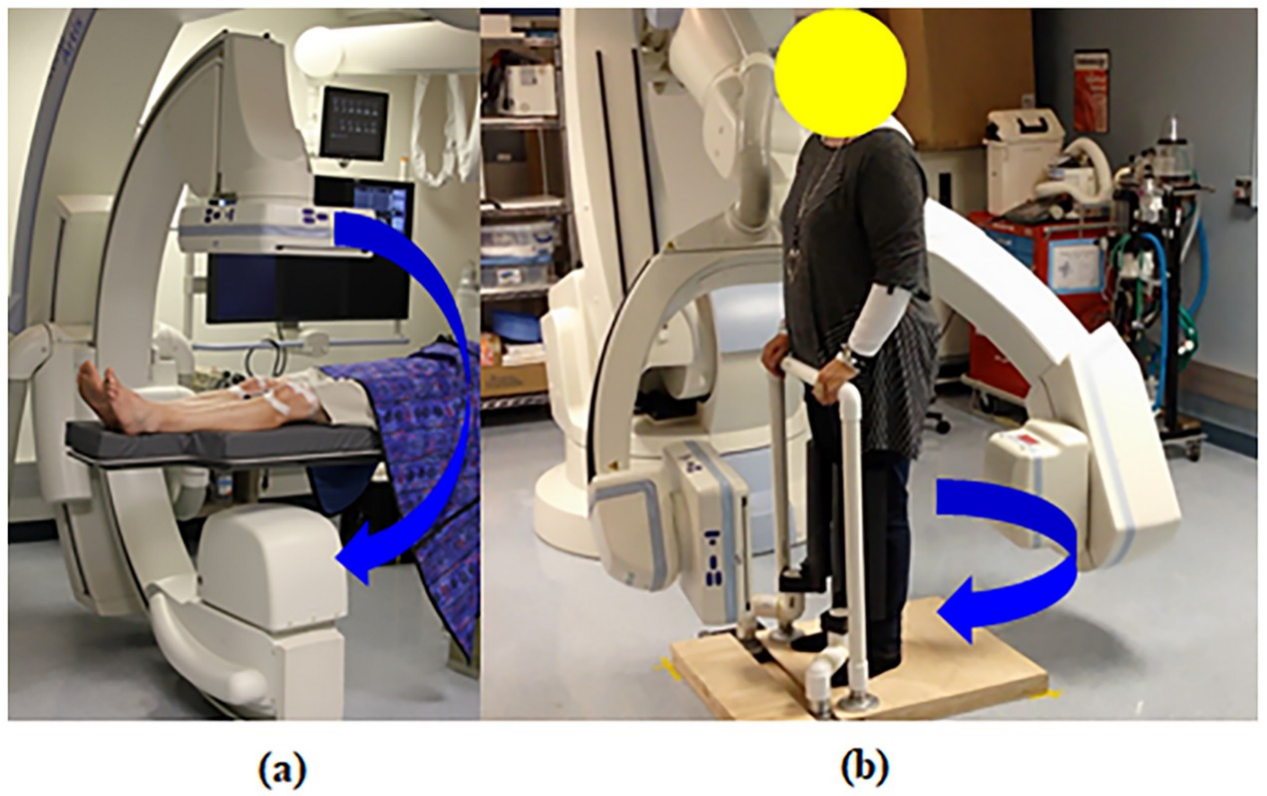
The C-arm CT system (a) in the supine (NWB) and (b) upright (WB) positions under the conditions of 0° and 30° knee flexion. (a) The detector and X-ray source rotated around the knee of the patient in the supine position. (b) The detector and X-ray source rotated around the knee of the patient in the upright posture.
